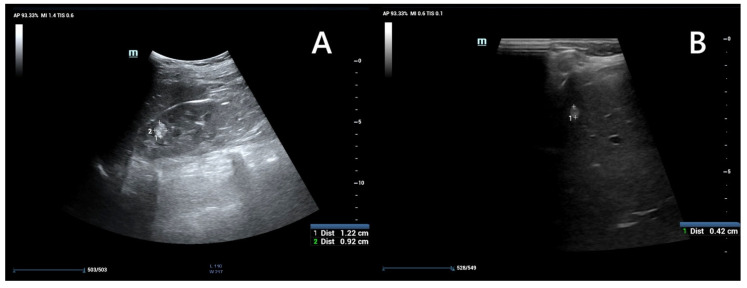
An ultrasound of a 13-year-old boy. Kidney with focal lesions with features suggestive of AMLs (A). Multiple oval hyperechoic lesions within the liver—most likely AMLs (B).
